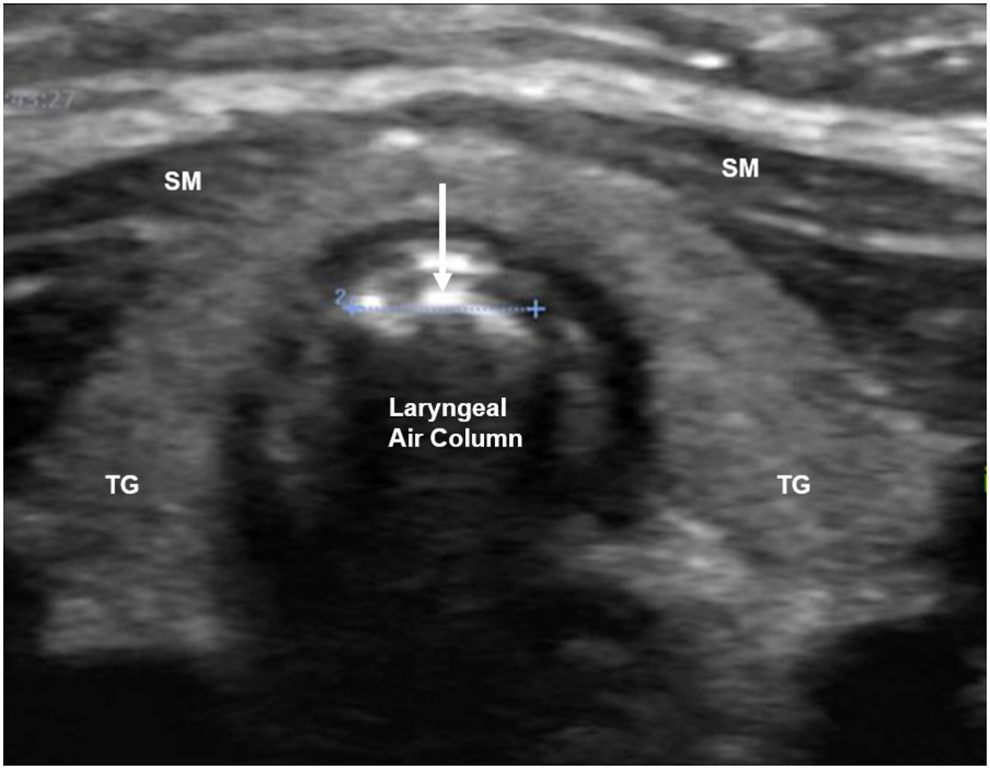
Airway ultrasound image at the level of thyroid gland demonstrating laryngeal air column width (arrow). TG, thyroid gland; SM, strap muscles.
